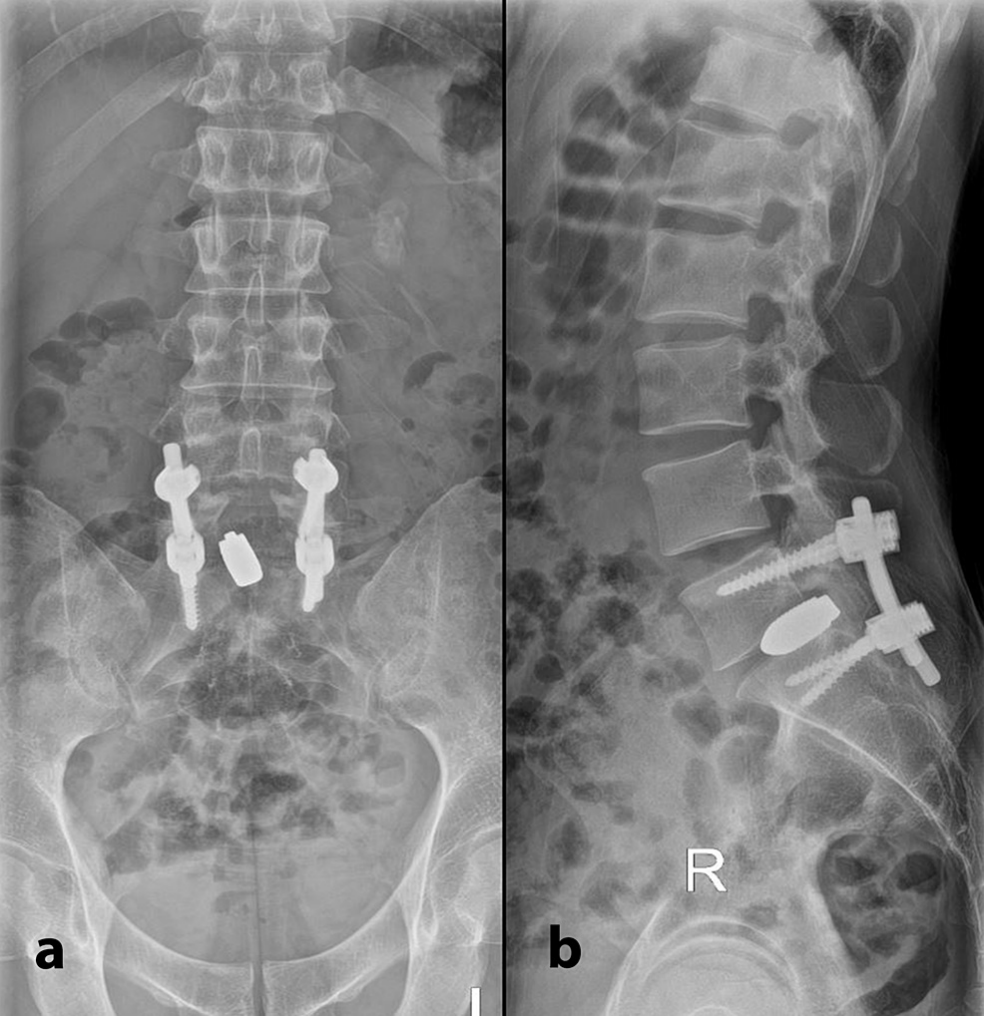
Antero-posterior (a) and lateral view (b) radiograph showing posterior instrumentation and malposition of tantalum cage protruding more than 50% into the spinal canal.
